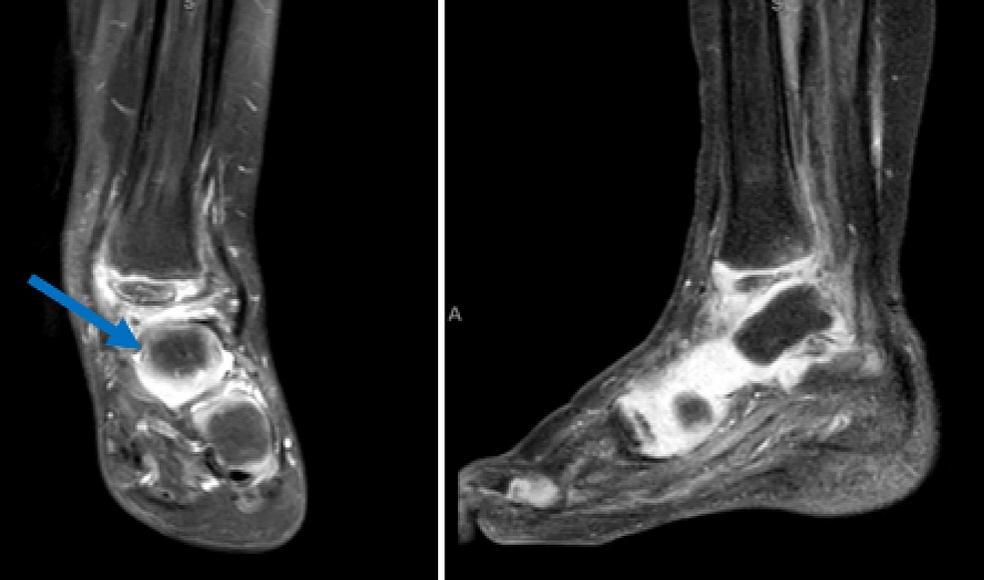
MRI demonstrating deformity A magnetic resonance imaging study of the left ankle demonstrates superolateral subluxation of the talus relative to the distal tibia in the coronal plane (Panel A) and sagittal plane (Panel B).
